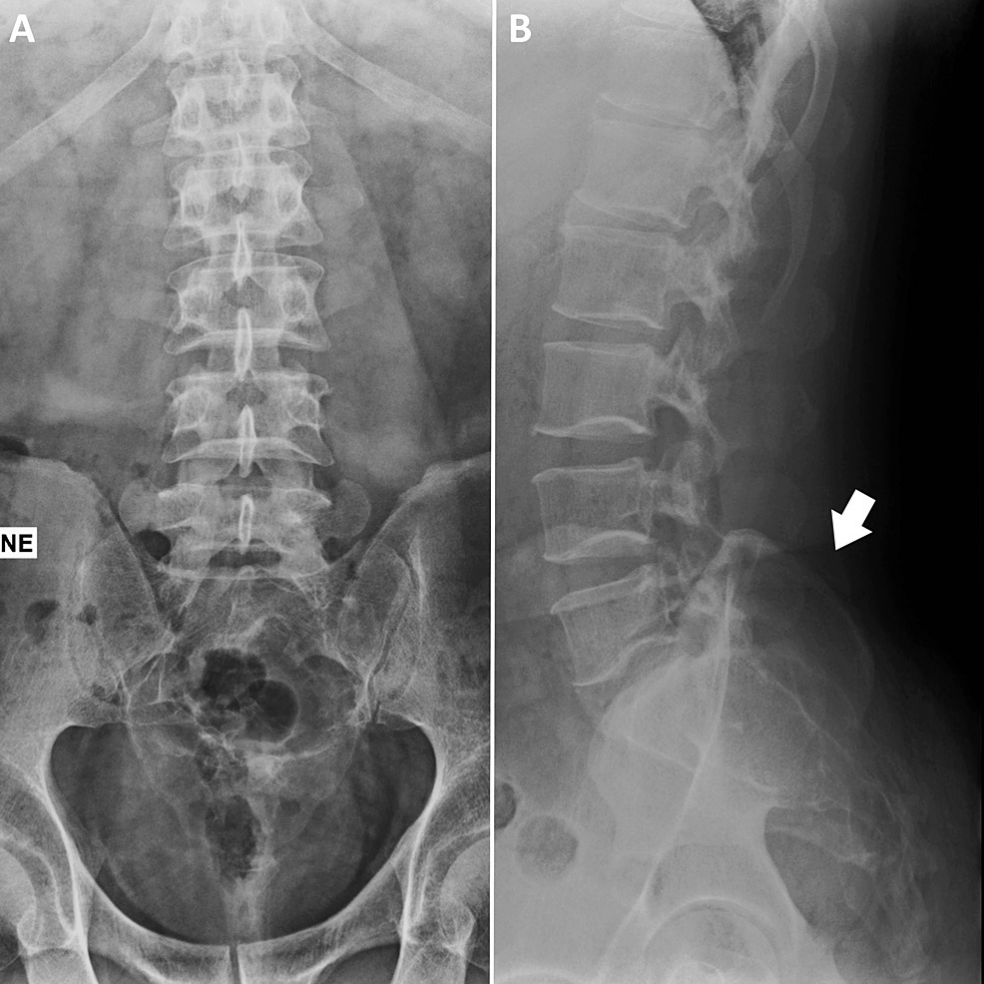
Lumbar radiographs. An expansile lytic lesion is identified at the sacrum (white arrow) in both (A) anterior-posterior and (B) lateral views of the lumbar radiographs.
